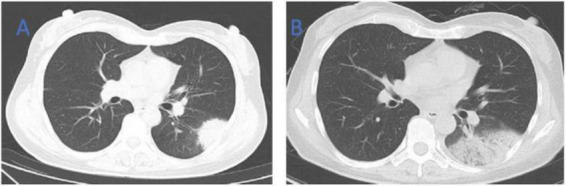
Chest CT of patient 1. (A) Before treatment on September 7, 2022; (B) the follow-up imaging 1 week after treatment showed progression compared to the pre-treatment scans.
